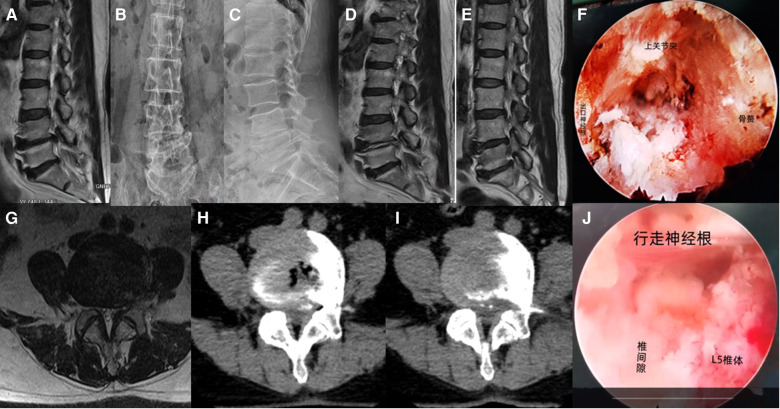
(A) preoperative MRI in sagittal position; (B,C) preoperative x-ray radiograph in lumbar dynamic position showing no lumbar instability or spondylolysis, the left L4/5 space collapsed and osteophytes formed; (D) sagittal MRI 2 days after surgery; (E) sagittal MRI 6 years after surgery; (F) the situation under the endoscope when inserting the working channel; (G) preoperative MRI in coronal position; (H) preoperative CT in coronal position; (I,K) coronal CT 2 days after surgery; (J) sufficient decompression of the nerve root was seen under the endoscope.
